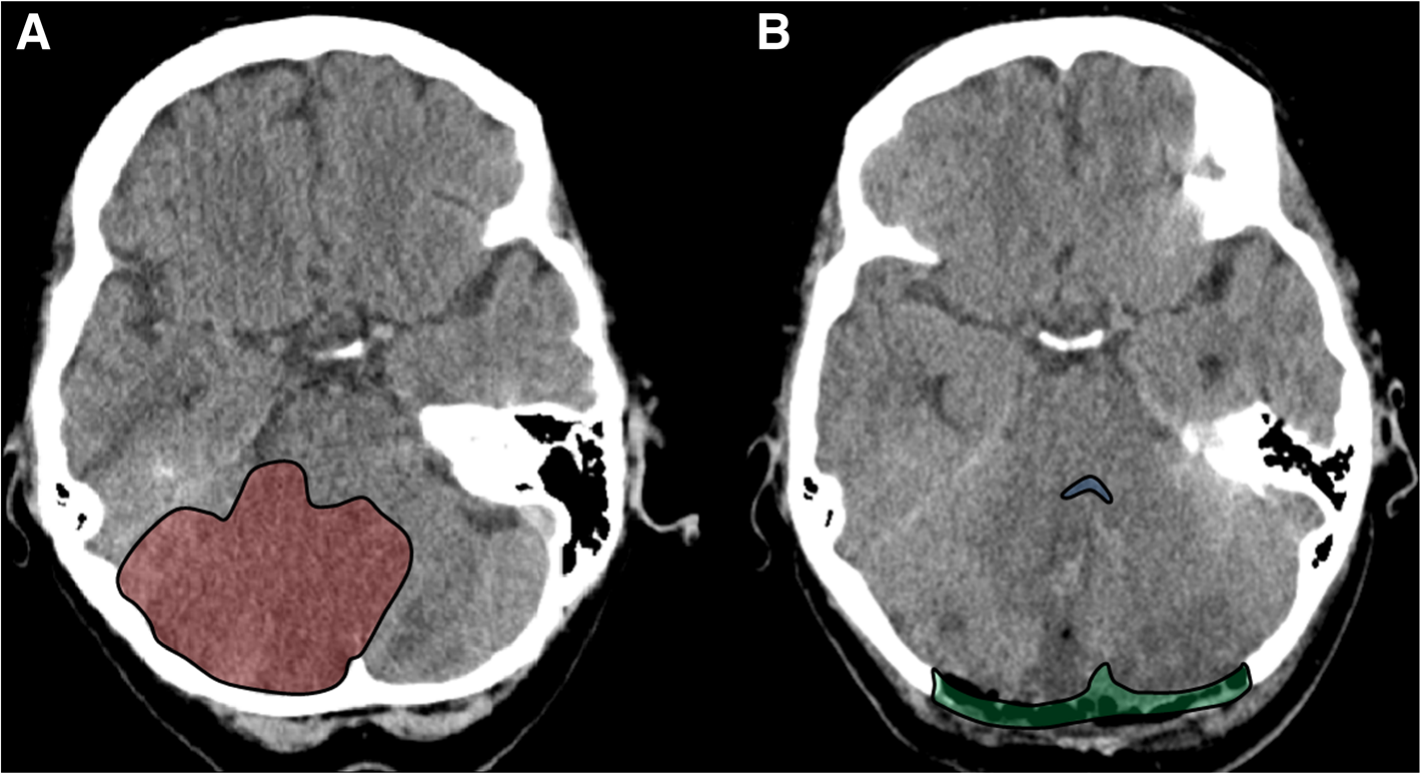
Suboccipital decompressive craniectomy for malignant cerebellar stroke. Axial CT scan before surgery (a), showing a large demarcated cerebellar infarct (highlighted in red). Axial CT scan after surgery (b), demonstrating the craniectomy defect (highlighted in green) and decompressed fourth ventricle (highlighted in blue)
